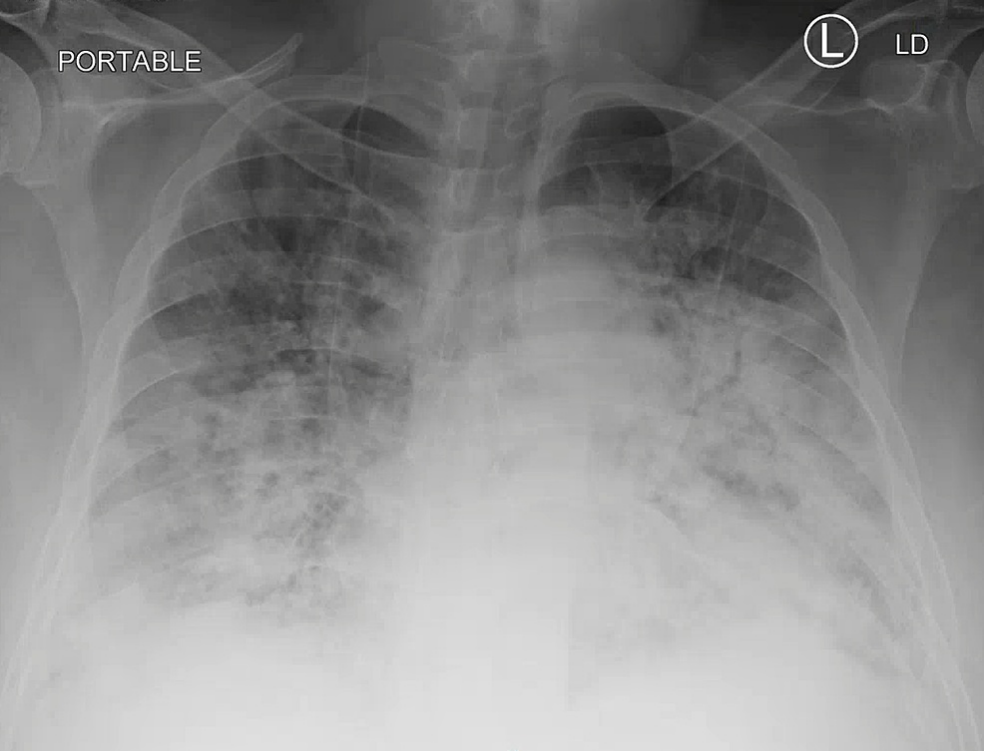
Chest X-Ray 1 Day Prior to Intubation
